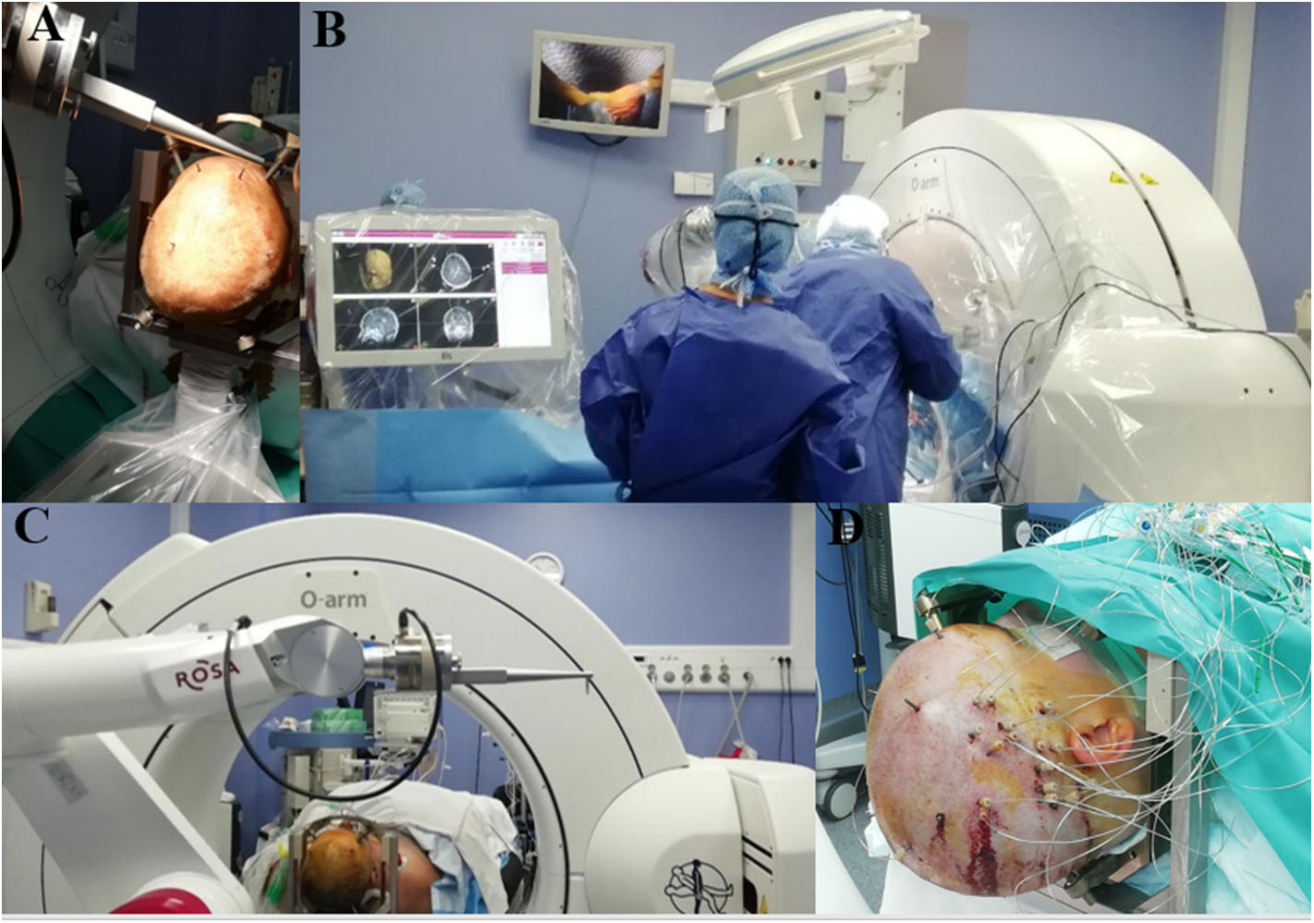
Example of ROSA-robot system in the operating room in Grenoble. (A) Five bone fiducials markers anchored in the patient skull in non-coplanar manner. (B) ROSA-robot system with sterile touchscreen easy to use interface and intraoperative CT. (C) ROSA-robot dedicated bone fiducials markers system for referencing process. (D) Immediate postoperative view of a right SEEG.
